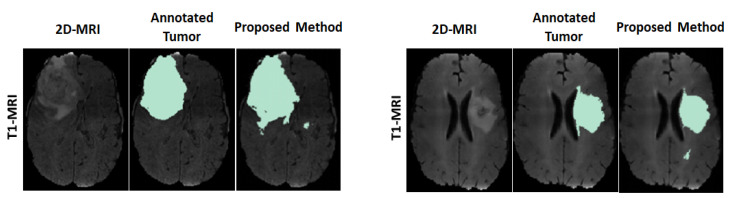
Example of two segmented brain tumor images from the MICCAI test set. Columns (on left and right): original T1 MR image; annotated tumor area marked by medical experts; segmented tumor area from proposed method.
